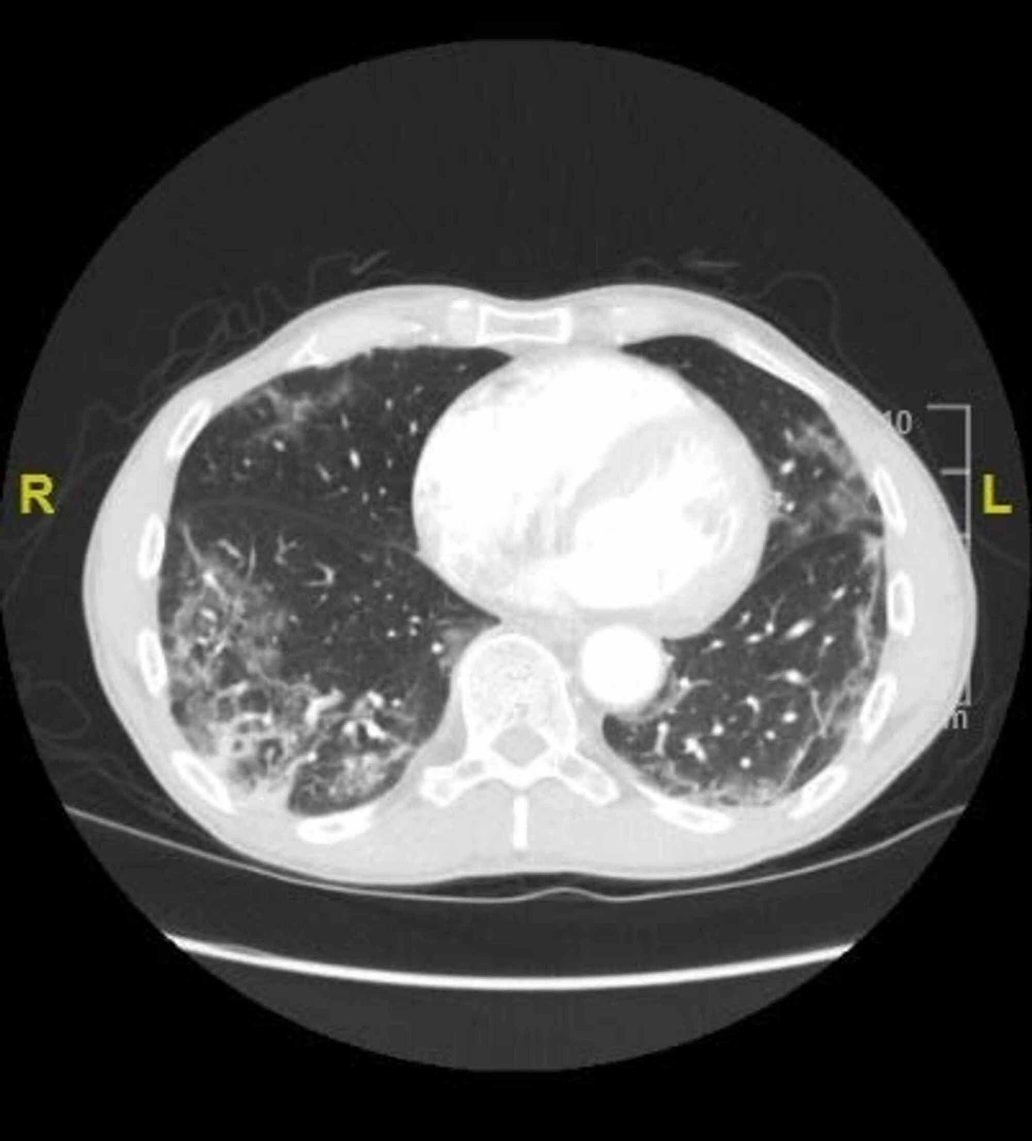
Cross-section of the CT-chest revealing extensive peripheral patchy ground glass opacities
